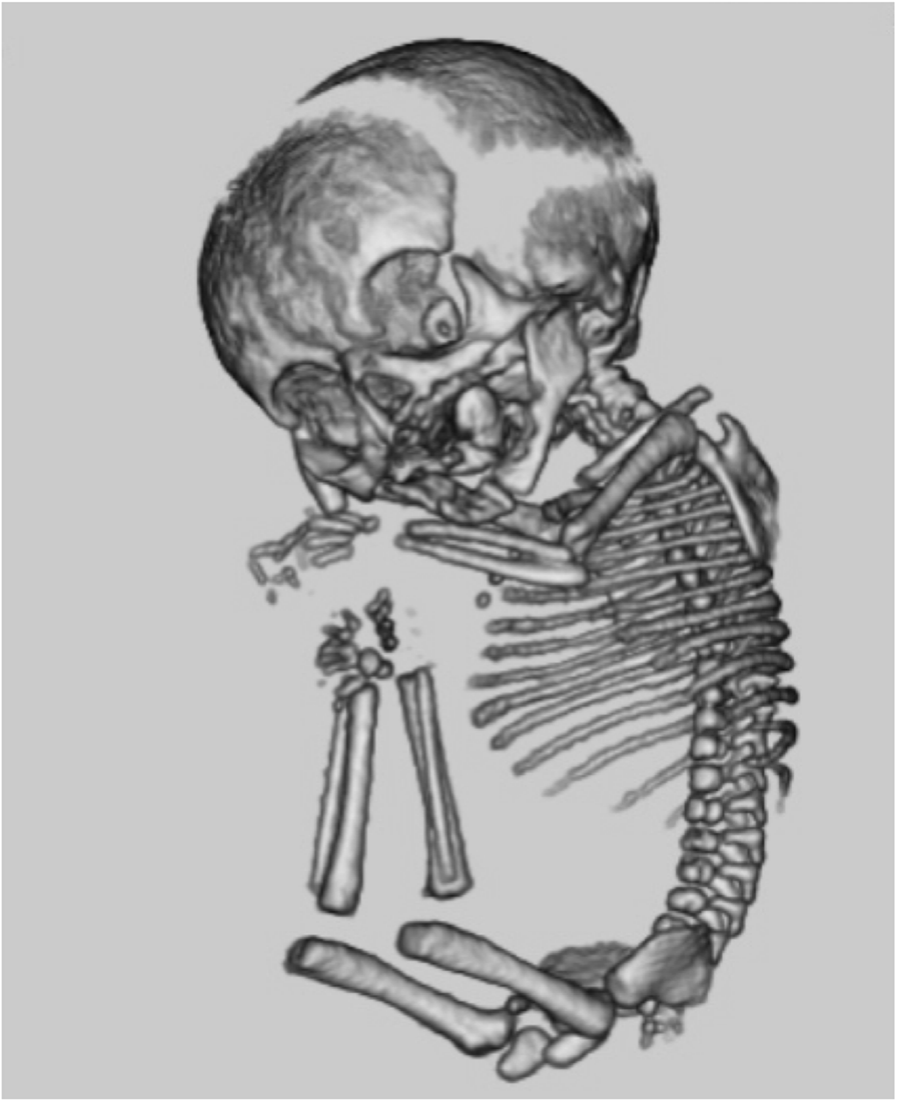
Fetal 3-dimensional computed tomography imaging of Infant 14: A case of Desbuquois dysplasia
